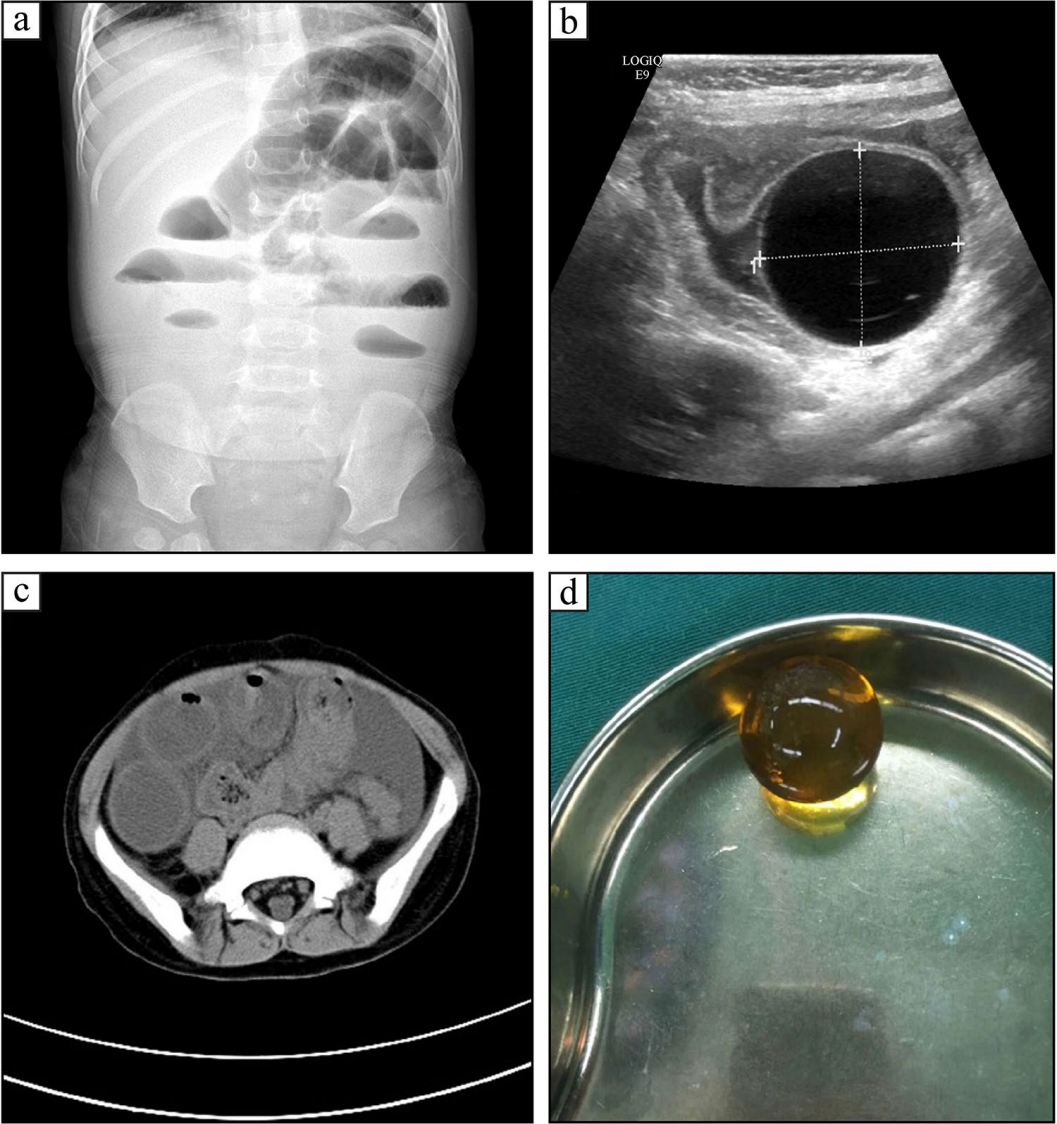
Water-absorbing bead ingestion. a: Abdominal radiographs indicating complete intestinal obstruction; b: Ultrasonography revealing a well-marginated hypoechoic mass in the intestinal cavity, with proximal intestinal dilatation; c: CT scanning showing intestinal obstruction without FBs detected; d: The water-absorbing bead was removed by surgery. *CT* computed tomography
